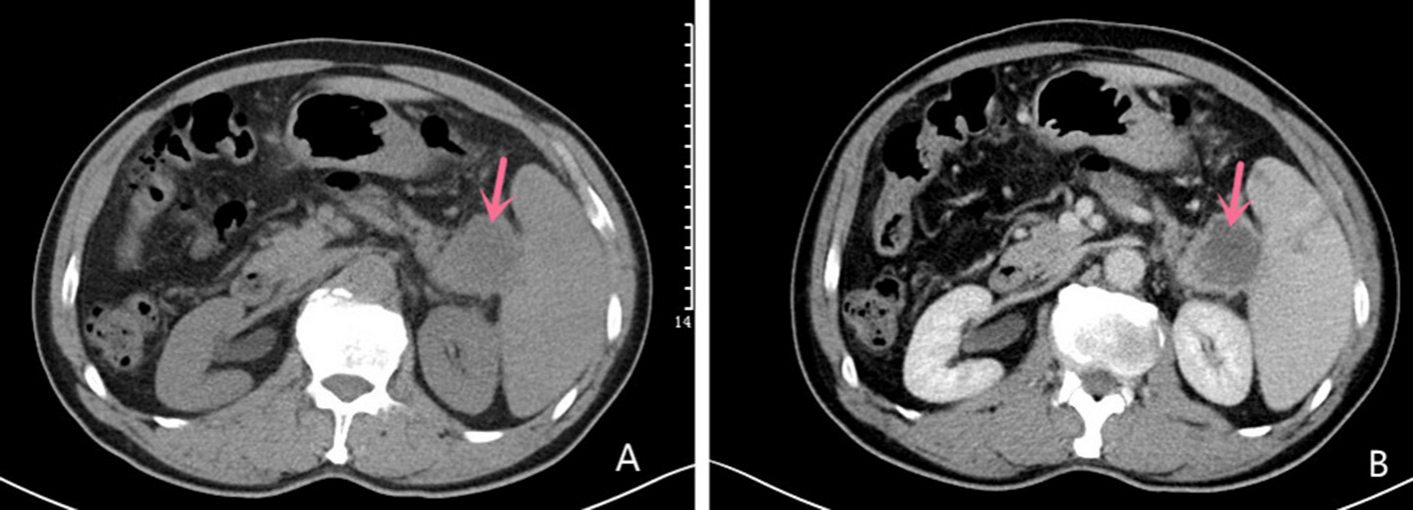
Computed tomography Imaging Computed tomography scan showing a mixed solid and cystic hypodense mass in the tail of the pancreas measuring 35 × 37 mm in the maximum diameter (A); Contrast-enhanced CT imaging showing the mass with obvious enhancement (B).
